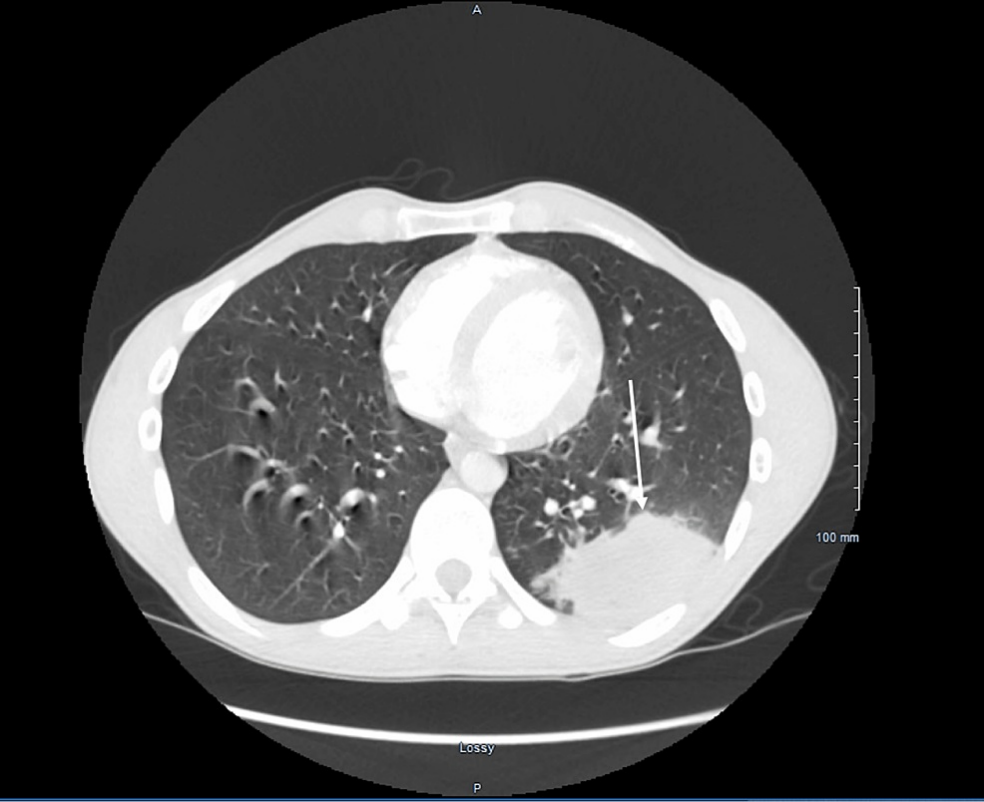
Transverse section of a Computed Tomography showing a 3.9 x 5.5 x 6.3 cm mass-like consolidative change in the left lung
